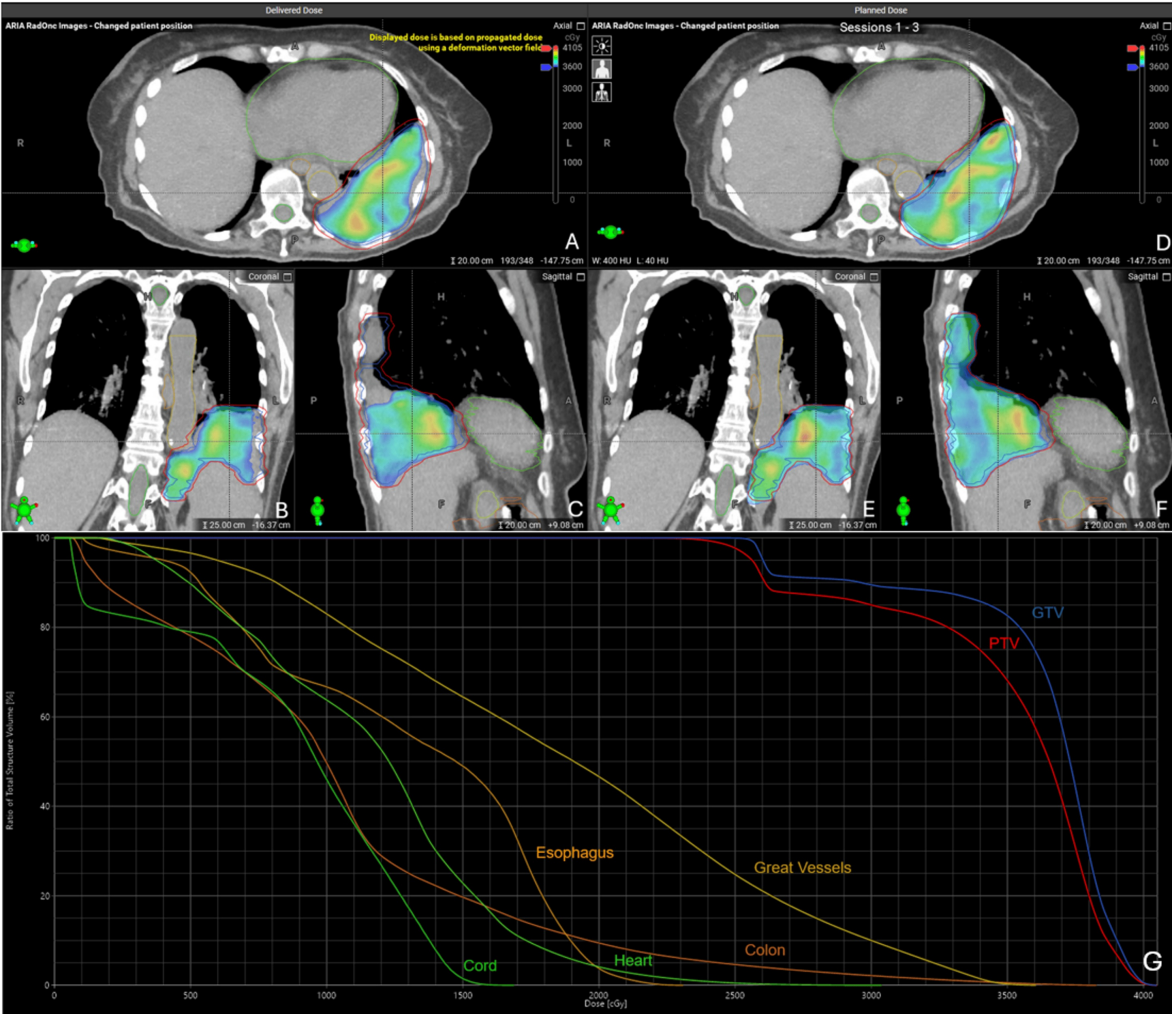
Plan Summary of Online Adaptive Radiotherapy Radiation summary showing delivered and planned dose colorwash overlaid on original planning computed tomography (pCT) scan. Delivered dose is displayed as an accumulation of dose from three fractions displayed on axial (A), coronal (B), and sagittal (C) pCT views. For comparison, the planned total dose is displayed onto the pCT on axial (D), coronal (E), and sagittal (F) views. Cumulative dose-volume histogram (DVH) from three delivered adaptive stereotactic body radiotherapy (SBRT) fractions (G). Contours from original reference plan are displayed with associated DVH curves for gross tumor volume (GTV; blue), planning target volume (PTV; red), great vessels (gold), colon (orange), esophagus (brown), heart (light green), and spinal cord (dark green).
